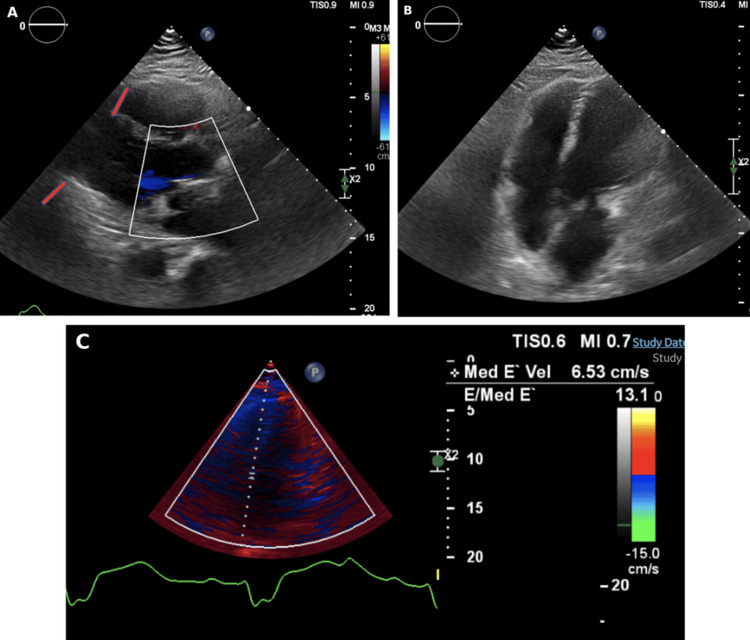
Transthoracic echocardiography. (A) Parasternal long-axis view; arrows indicate the moderately increased left ventricular wall thickness. (B) Apical four-chamber view showing normal left ventricular chamber size and moderate left atrial dilation. (C) Tissue Doppler imaging with a medial (septal) E/e′ of 13.1, consistent with elevated left ventricular filling pressure.
